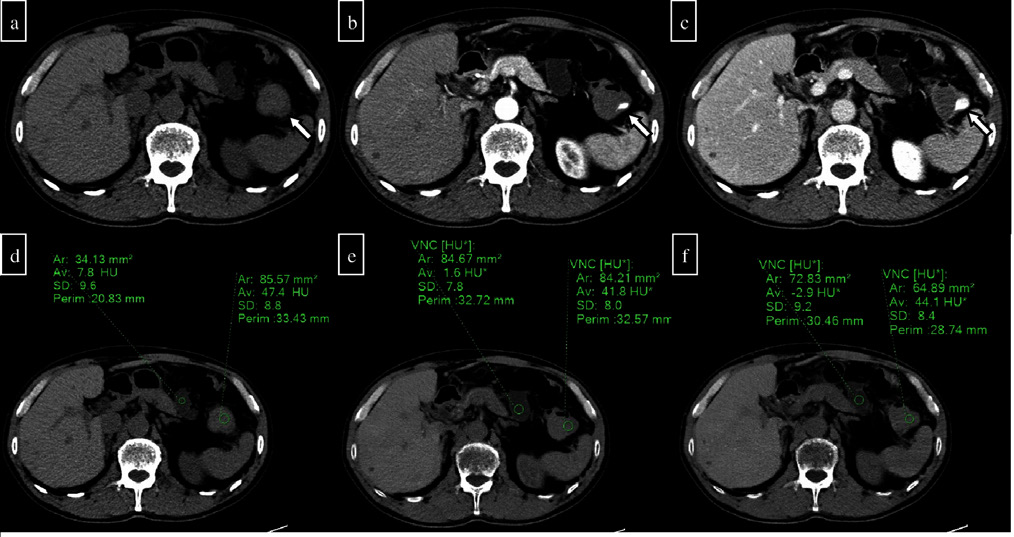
A 70-year-old man presented to the clinic for bleeding after the endoscopic mucosal resection of a colonic polyp and underwent pre- and post-contrast two-phase CT (arterial and delayed phases). (a) Pre-contrast CT showed a relatively high density of contents in the splenic flexure of the colon, thus suggesting a haematoma (arrow). (b) The arterial phase shows extravasation in the splenic flexure. (c) Extravasation is increasing in the delayed phase. From the above, active haemorrhage after endoscopic mucosal resection was suspected. (d) Pre-contrast CT measurement shows a 47.4 HU in the splenic flexure lumen and a 7.8 HU in the small intestine lumen in the area nearby. (e) In the VNC created from arterial phase images, the densities of the splenic flexure and the small intestine in the nearby area are 41.8 and 1.6 HU, respectively. (f) In the VNC created from the delayed phase image, the densities in the splenic flexure and the small intestine in the nearby area are 44.1 and -2.9 HU, respectively. There is no significant difference that would quantitatively affect the evaluation. VNC, virtual non-contrast CT.
